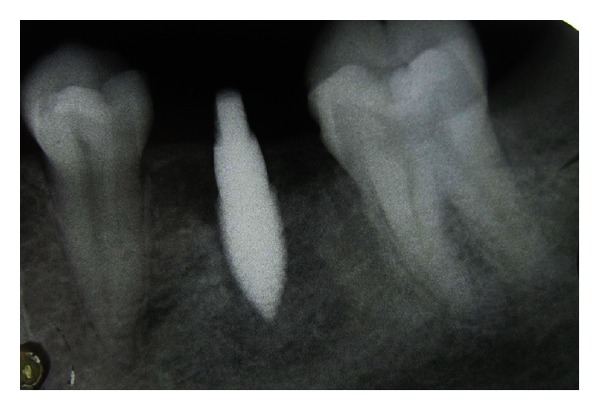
Radiographic view of the implant after surgery.
